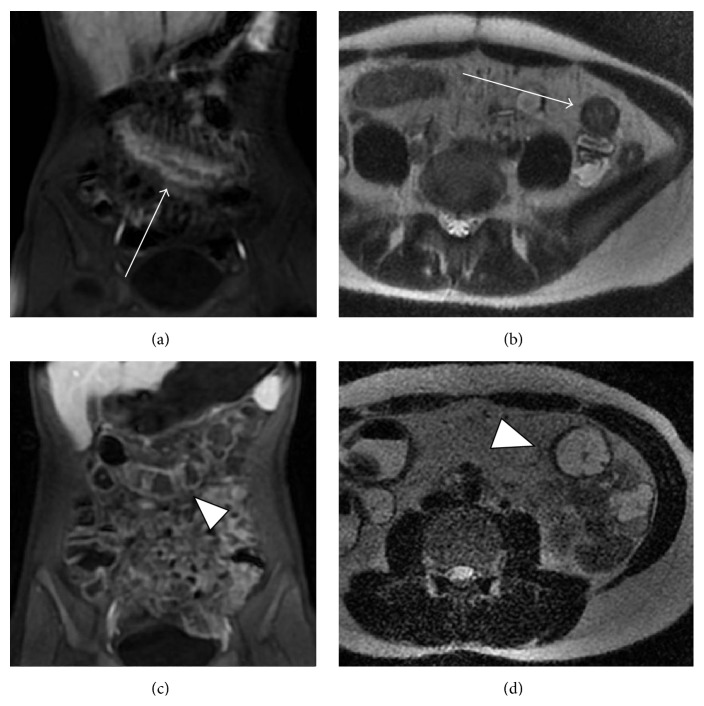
Mucosal healing on serial MRE in a patient with Crohn's disease affecting the transverse colon. (a) Coronal T1-weighted postgadolinium with fat saturation and (b) axial T2-weighted images demonstrating bowel wall thickening, mural edema, mucosal hyperenhancement, and the comb sign (arrows) on initial imaging. Subsequent MRE performed after treatment ((c) and (d)) demonstrates normalization of imaging findings (arrowheads) correlating with mucosal healing at endoscopy.
